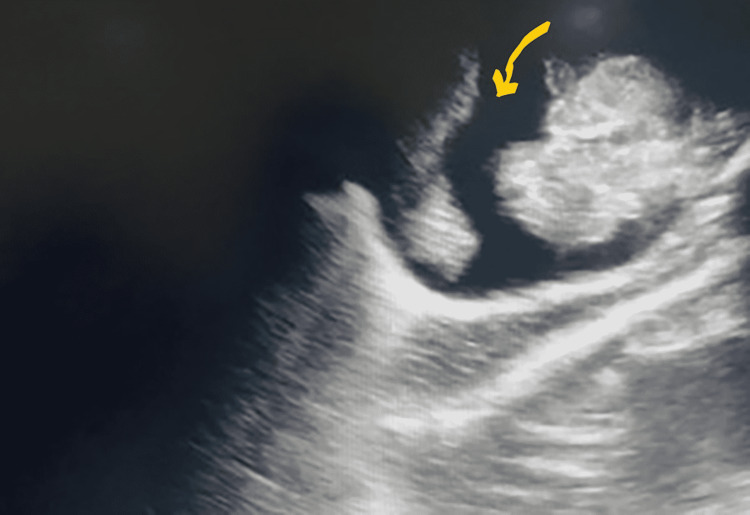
Ultrasound showing severe ascites
